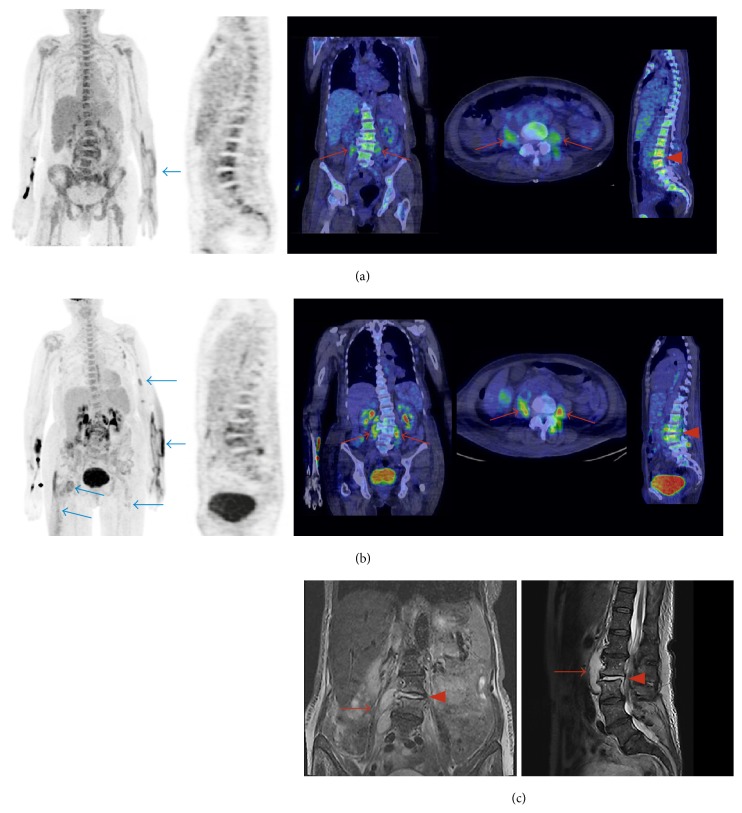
Patient #1 was a 66-year-old woman (weight: 65 kg) who presented at the hospital because of back pain and general symptoms. Both ^68^Ga-citrate (a) and ^18^F-FDG PET/CT (b) showed vertebral osteomyelitis (spondylodiscitis;* red arrowheads*) and abscesses in the iliopsoas and paravertebral area (*red arrows*). These were confirmed by MRI (c). ^18^F-FDG PET/CT also showed other multiple soft tissue infectious foci ((b),* blue arrows*), some of which were not detectable on ^68^Ga-citrate PET/CT ((a),* blue arrow*). The injected radioactivity dose of ^18^F-FDG was 227 MBq and the PET acquisition started 54 min after injection. The injected radioactivity dose of ^68^Ga-citrate was 245 MBq and the PET acquisition started 88 min after injection. MRI sequences were as follows: T2-weighted short inversion time inversion recovery (STIR) on the coronal view image (left) and T2-weighted on the sagittal view image (right).
